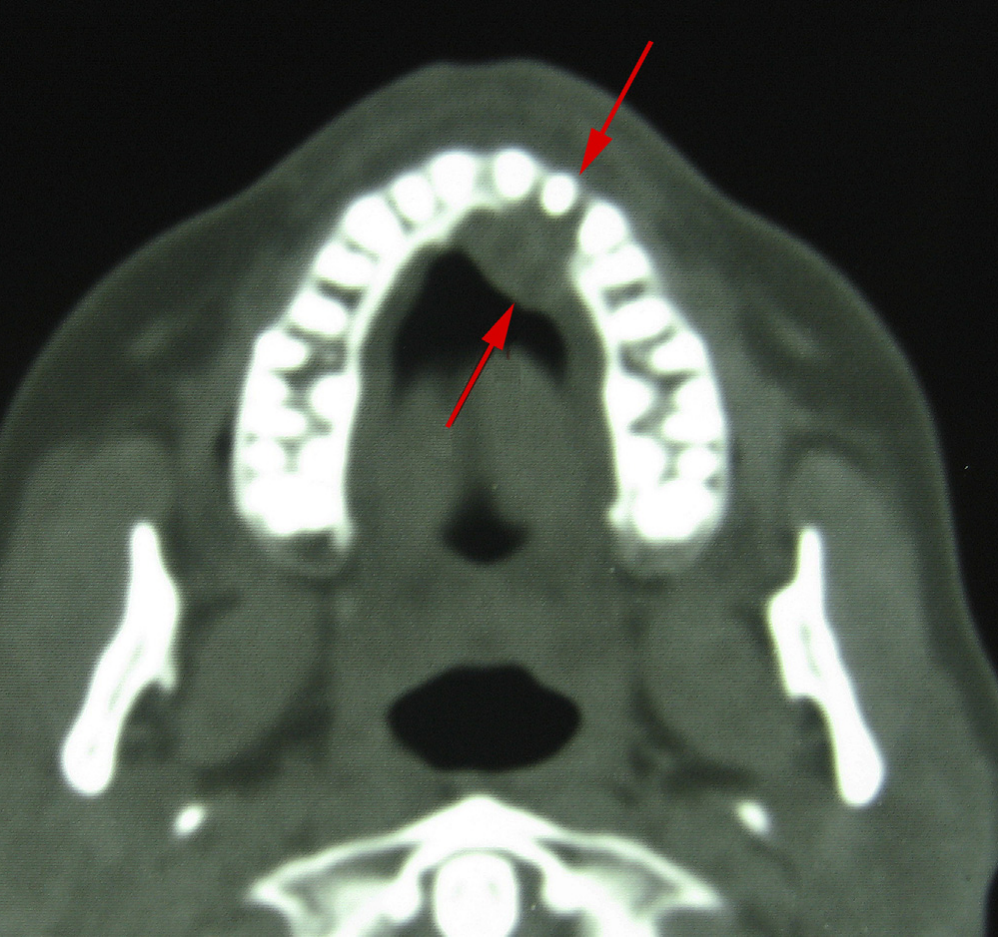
Axial CT scan of the maxilla showing the tumour and destruction of the alveolar process palatinal to tooth 22 (Arrow).
